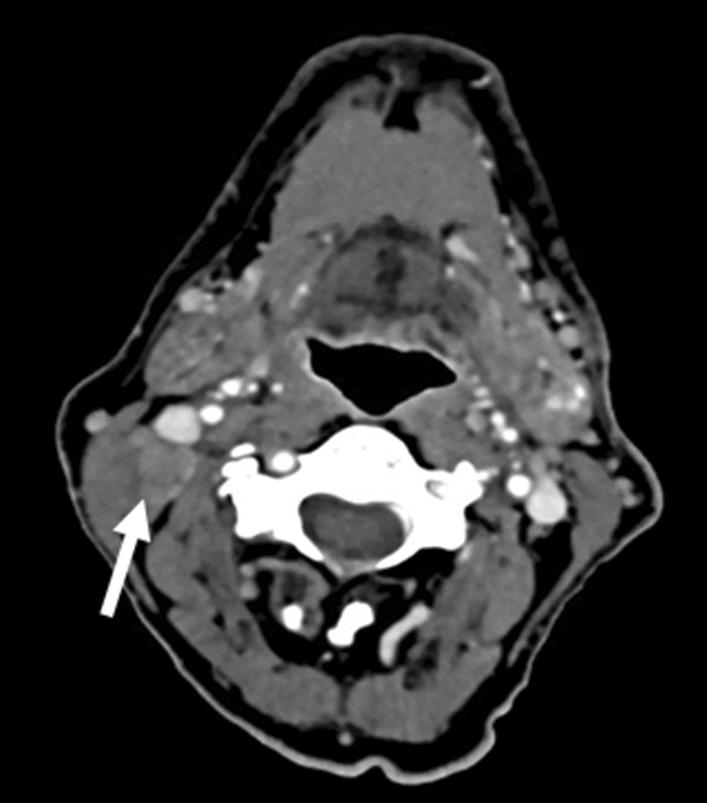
CT scan of a patient with a base of tongue OPSCC with right cervical lymph node metastasis with clinical features of extranodal extension which were confirmed by pathology (white arrow)
